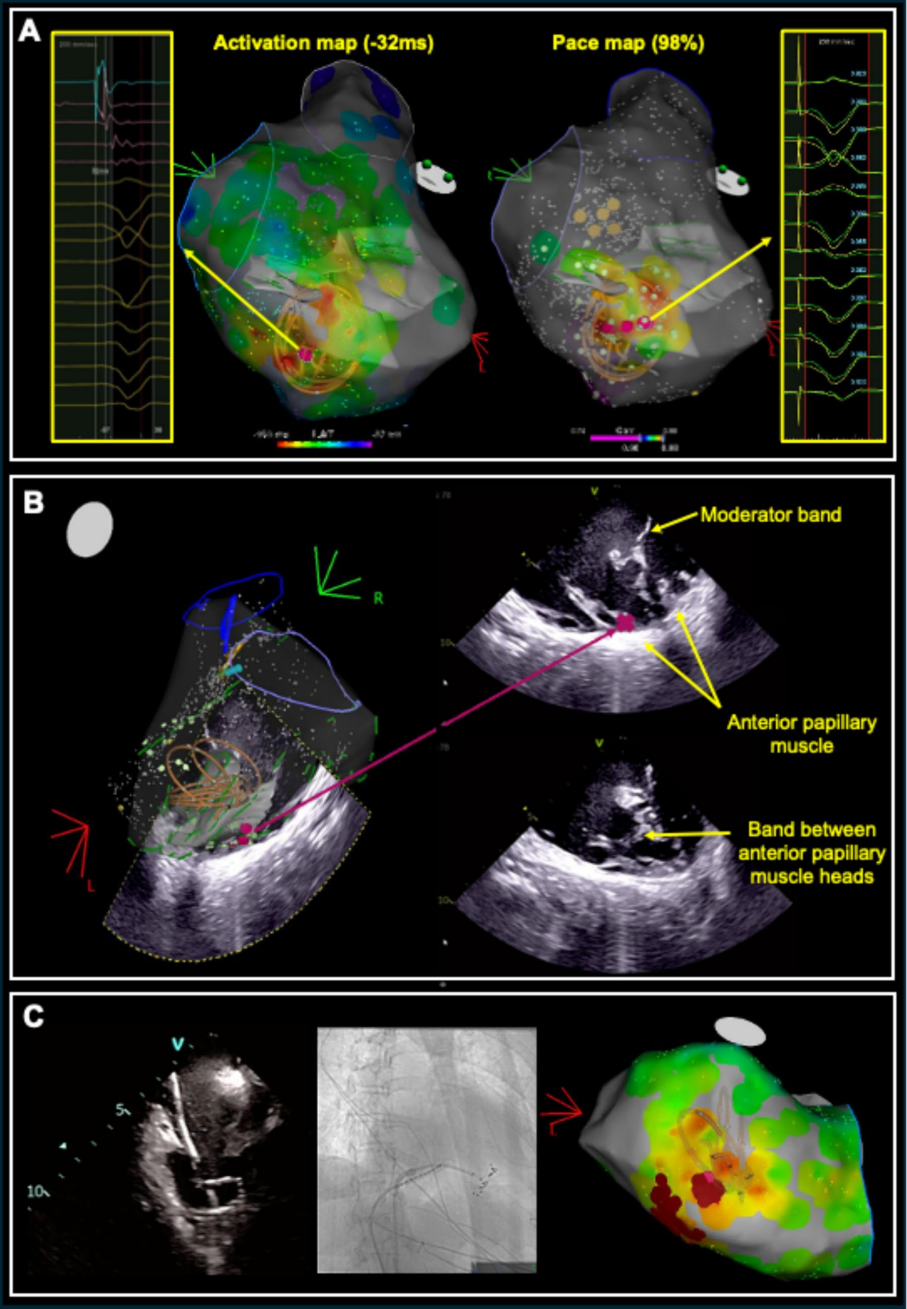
Case 2: A Activation and pace map demonstrates localisation of the PVC to the right ventricular papillary muscle (pink dot is best site). The map has been made transparent to demonstrate location of PFA catheter (shown on this electroanatomic map as a lasso-shaped virtual catheter). B Intracardiac echocardiography (ICE) demonstrates a complex right ventricular papillary muscle (band noted between two heads), localising the region to the base of the muscle. C ICE demonstrates manoeuvring of PFA catheter in “basket” formation into the RV apex. Fluoroscopy (right anterior oblique 30) demonstrates difficulty to advance catheter further to apex. Electroanatomic map demonstrates adjuvant lesion delivery with radiofrequency ablation
